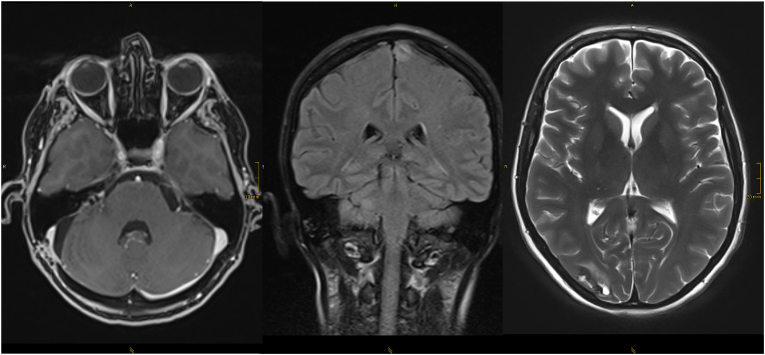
Follow up MRI (T1 Vibe post contrast axial reconstruction, coronal FLAIR, axial T2w section, from left to right) two months after resection of the tumor showing complete resolution of the acute PRES with hemosiderin residues visible at the right hand side occipital pole in the T2w image.
